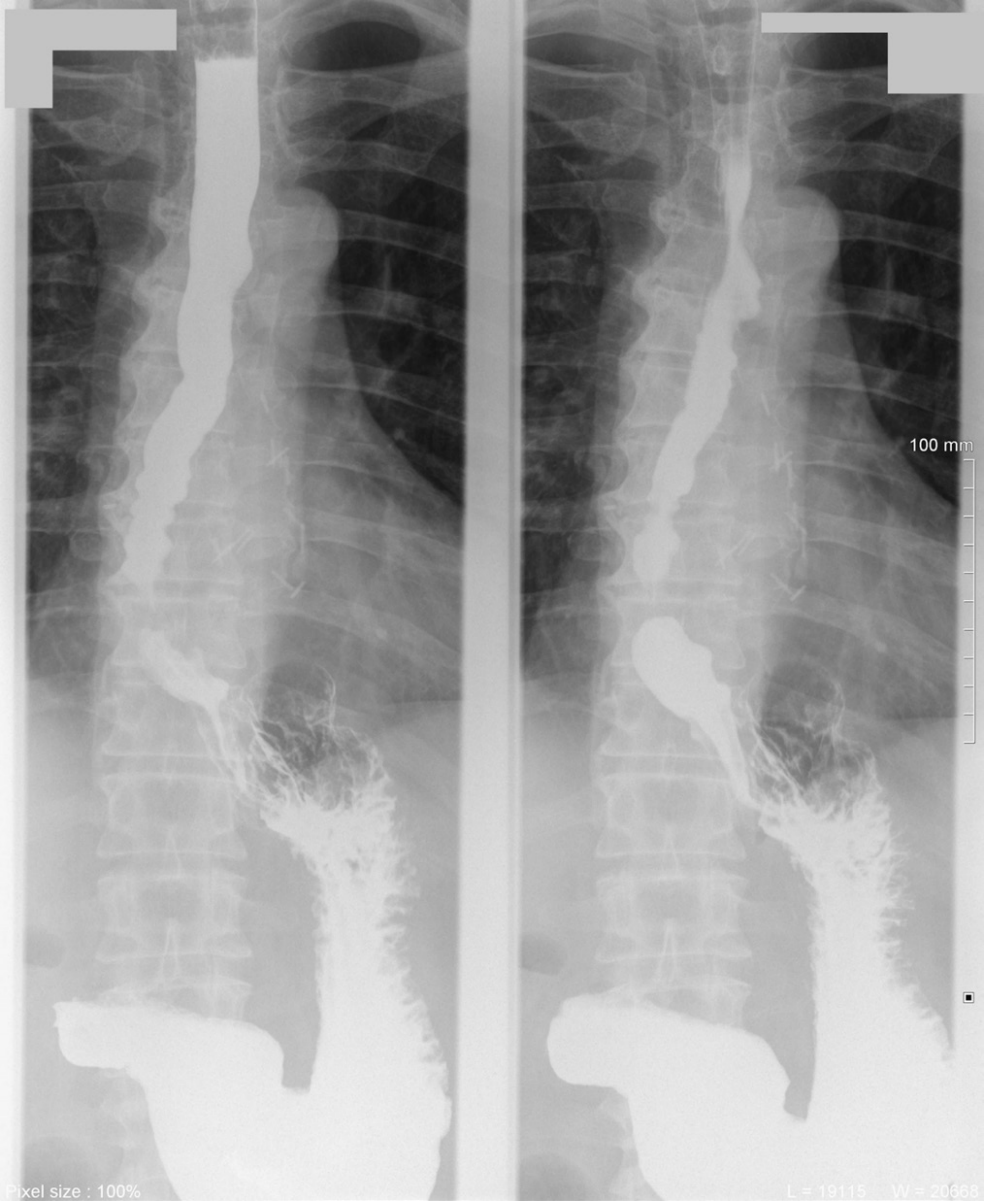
3 months post-surgery, Barium swallowing X-ray showed no recurrence of diverticulum.
